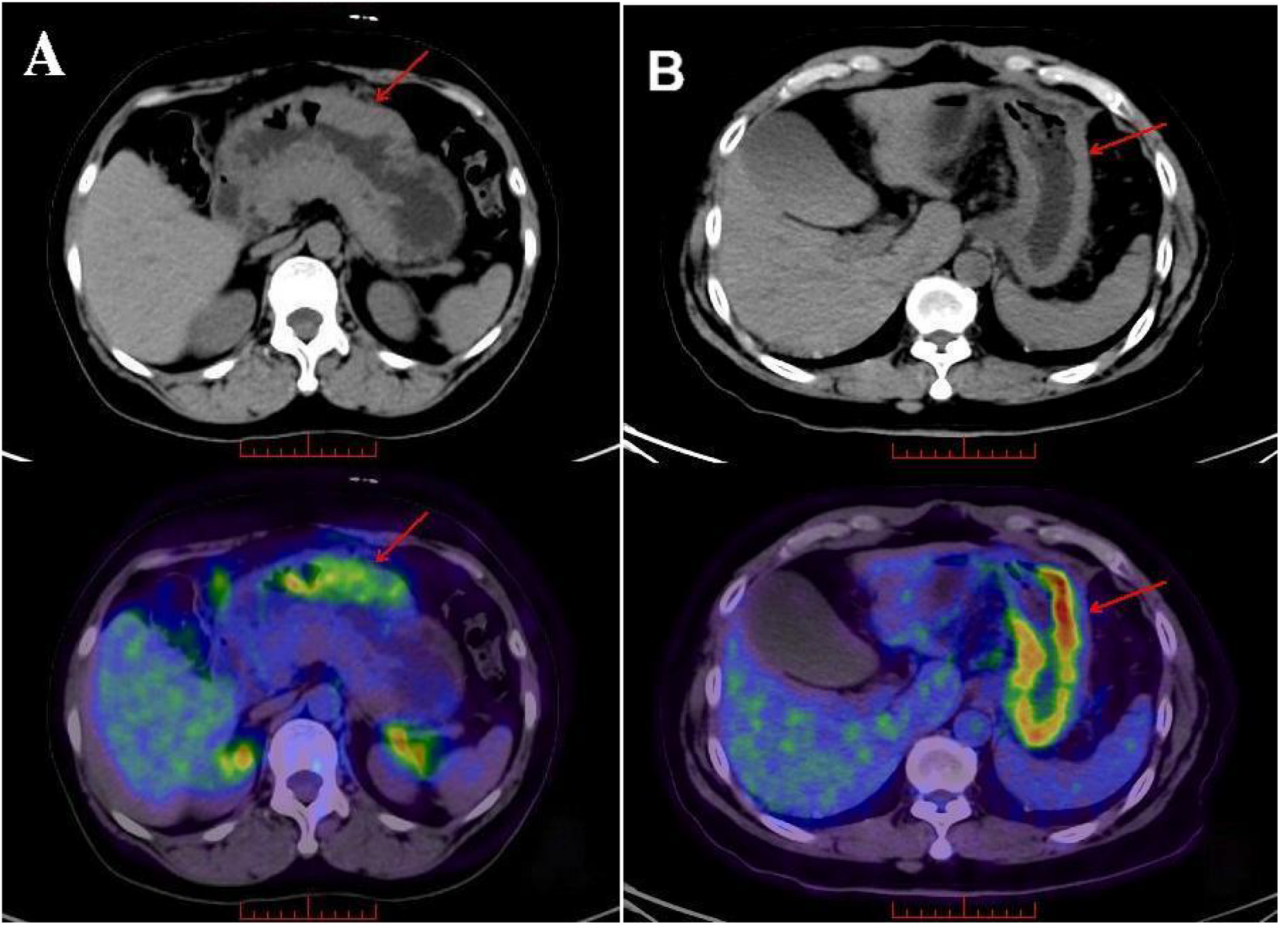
^18^F-FDG PET/CT images of HER2-positive (A) and HER2-negative (B) patients.
